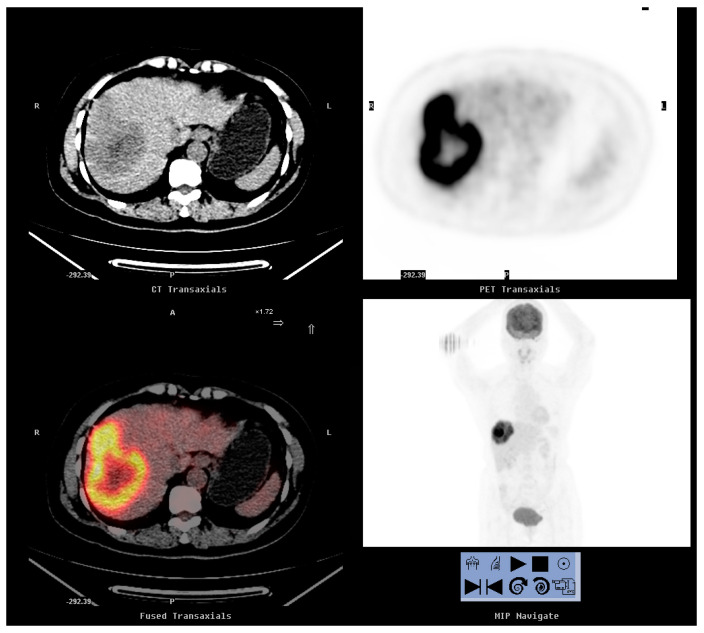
A 56-year-old woman who had cervical cancer (SqCC, FIGO stage IB1) 5 years previously presented with an elevation of the serum SCC-Ag level to 1.8 ng/mL. FDG-PET/CT revealed an 11.0 cm FDG-avid hypodense nodule in the right hepatic lobe (SUVmax: 7.9). The patient underwent right lobectomy, and pathology revealed a moderately differentiated metastatic SqCC, compatible with the findings of a tumor with cervical origin. However, the tumor relapsed, and her disease progressed. The patient died 3 years after the FDG-PET/CT examination.
